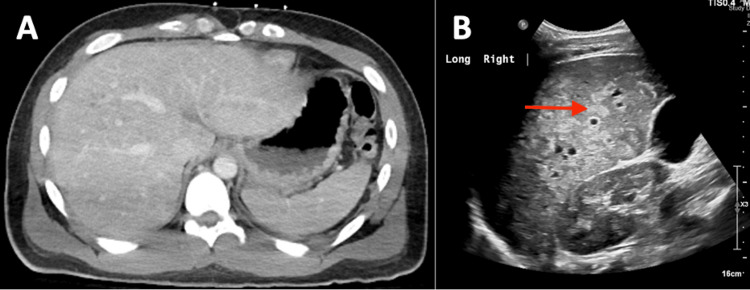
Hepatic Kaposi sarcoma (KS) on abdominal imaging Abdominal imaging revealed numerous subcentimeter lesions scattered throughout the liver representing probable hepatic KS. The lesions are very subtle on computed tomography (a) and more apparent on ultrasound (shown with red arrow) (B).
